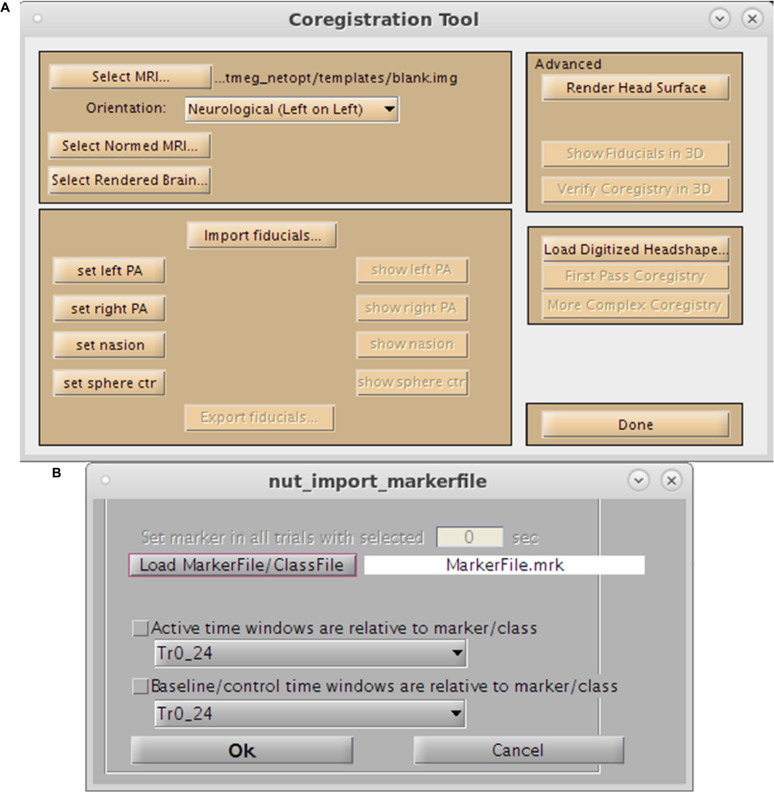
Preprocessing Toolbox GUIs. (A) Coregistration Toolbox GUI. Options include loading of the single-subject MRI, normalized MRI, fiducial assignment, importation of surface mesh, or headshape points. (B) Marker selection tool.
